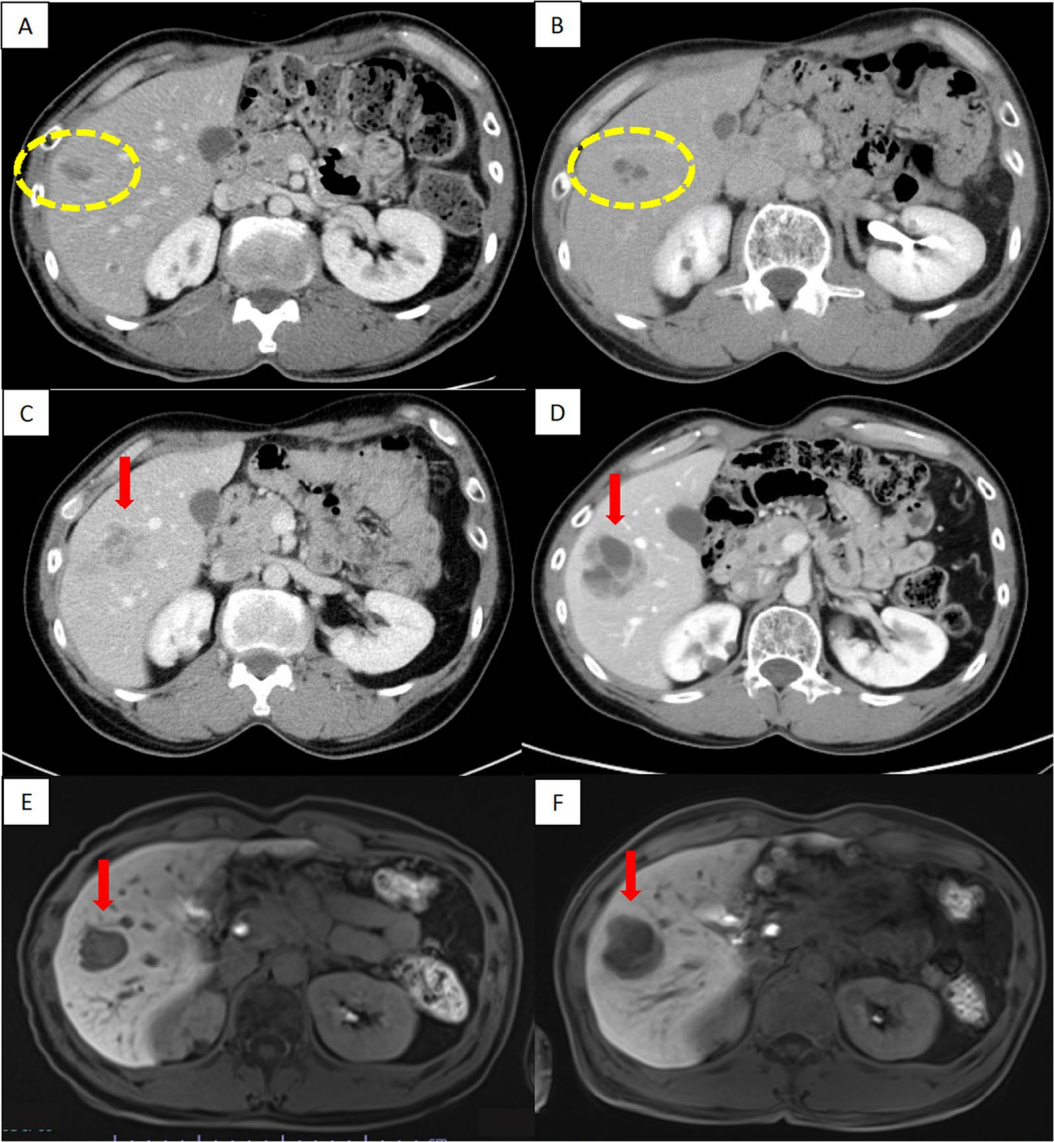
Enlargement of PNET liver metastasis during follow-up. A, B There was no obvious tendency toward enlargement at 6 months (A) and 6 years (B) after the initial surgery (circle). The greatest diameter increased from 23 to 36 mm after one year (arrow). C, D Contrast-enhanced CT scan. E, F Contrast-enhanced MRI (T1-weighted with fat suppression). *CT* computed tomography, *MRI* magnetic resonance imaging
